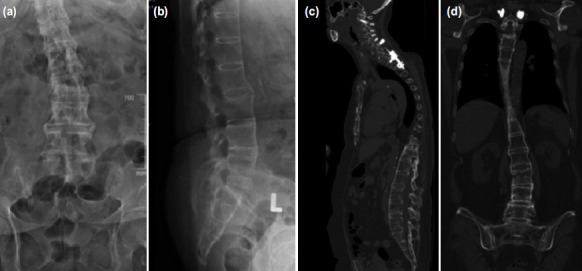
A 67-year-old lady with underlying ankylosing spondylitis presented with back pain and Frankel D neurology after a fall from standing height. Plain radiographs (a,b) and computed tomography (CT) scan (c,d) showed L3 hyperextension fracture. She has a history of C4/5 disc hyperextension fracture 2 years ago in which a long-construct posterior spinal fusion from C2 to T3 (9 instrumentation levels) was performed.
